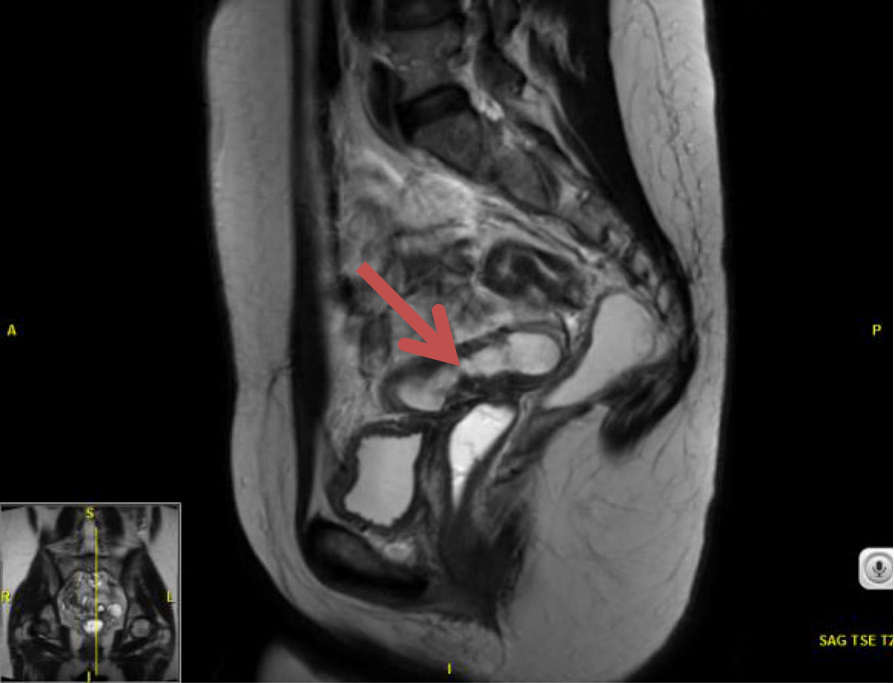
Sagittal T2-weighted magnetic resonance imaging section of pelvis with recto-sigmoid colon endometrial implant (*red arrow*)
